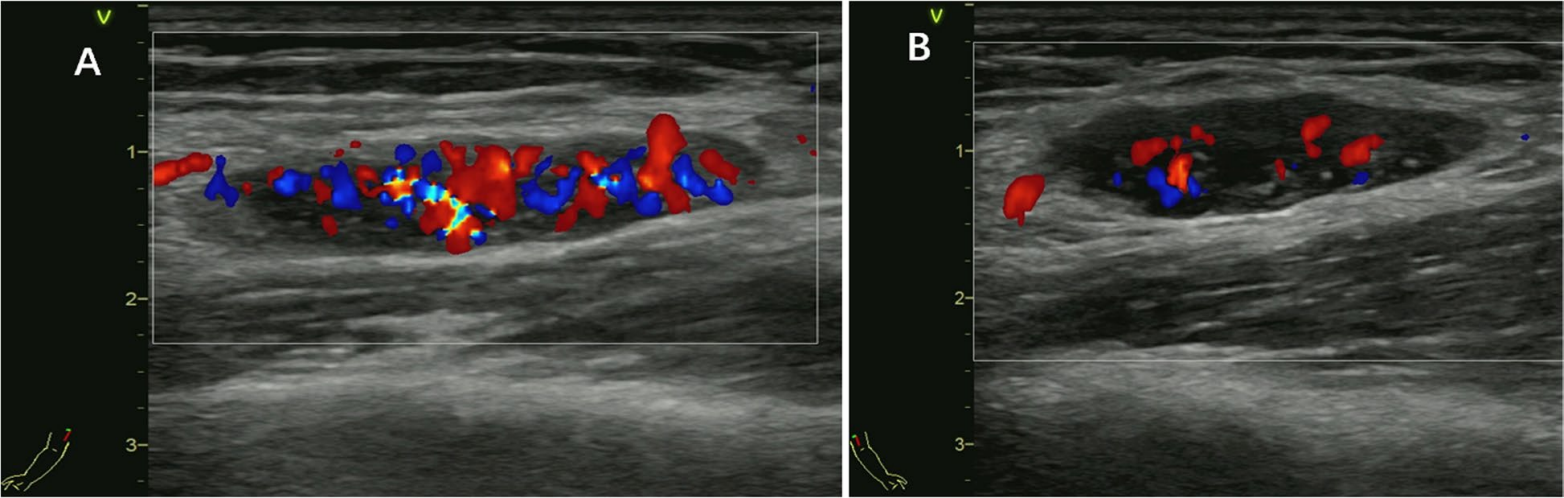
The Colour Doppler ultrasound of bilateral elbow. The bilateral elbow masses are oval in shape and have a heterogeneous hypoechoic-isoechoic appearance; the blood flows are relatively rich. (A is the right elbow; B is the left elbow)
